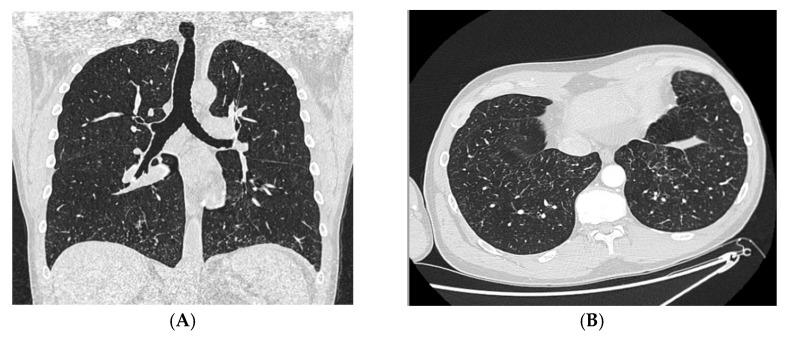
(A) HRCT coronal and (B) axial reconstructions demonstrating mild smooth thickening of the interlobular lung interstitium and the presence of many micronodules, especially in the basal sectors of the lower lobes.
